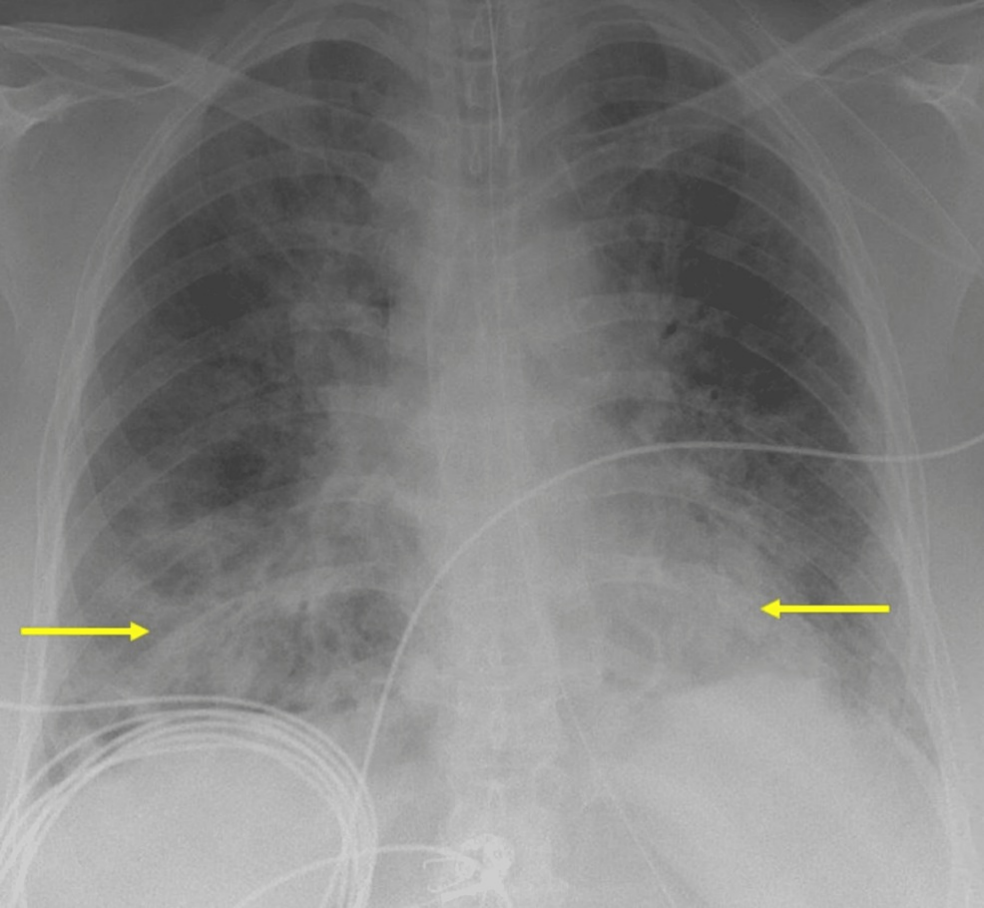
Chest X-ray demonstrating bilateral infiltrates suggestive of COVID-19 pneumonia (yellow arrows).
